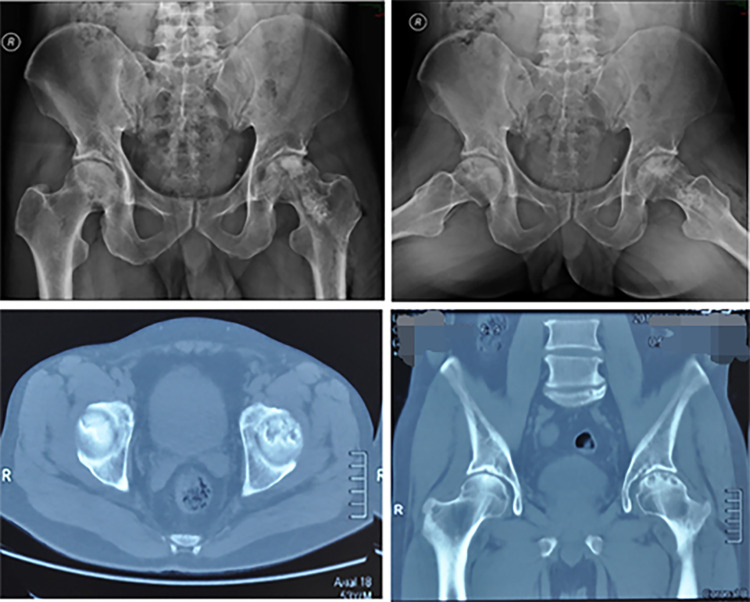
X-ray and CT image results of bilateral femoral heads obtained at the time of the first admission.
